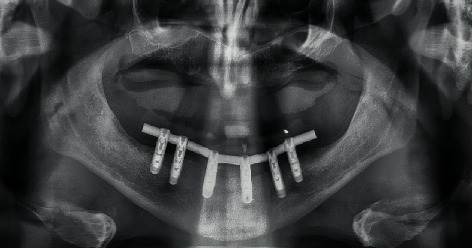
Radiographic panoramic control. After 6 years, bone level was stable.
